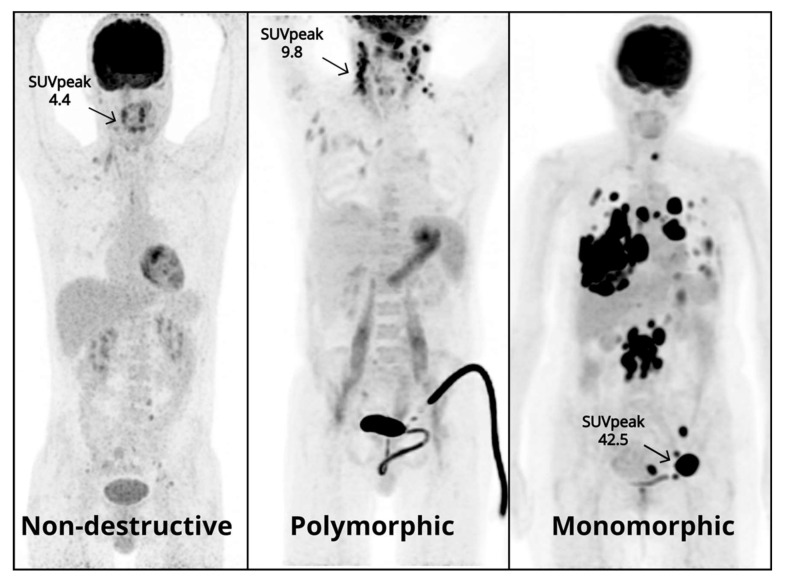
Representative PTLD patient for each morphological subtype with SUV_peak_ at biopsy site.
